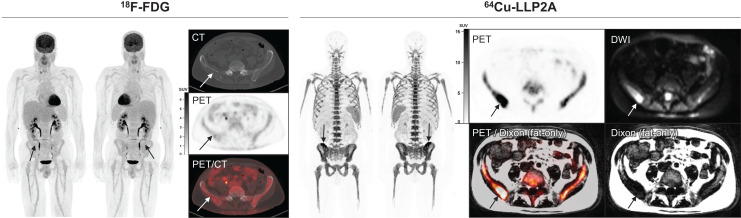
MM patient underwent PET imaging with ^64^Cu-LLP2A and ^18^F-FDG. On PET/CT, osteolytic lesion in right iliac bone (arrows) of MM patient had ^18^F-FDG uptake similar to background marrow. On PET/MRI, this same lesion (arrows) had ^64^Cu-LLP2A uptake above background marrow, corresponding to fat-replacing lesion on fat-only Dixon images and hyperintense lesion on DWI. In this lesion, ^64^Cu-LLP2A SUV_max_ was 29.5 with SUL_peak_ (per PERCIST) of 18.7; in comparison, ^18^F-FDG SUV_max_ was 2.9 with SUL_peak_ (per PERCIST) of 2.1. DWI = diffusion-weighted imaging.
